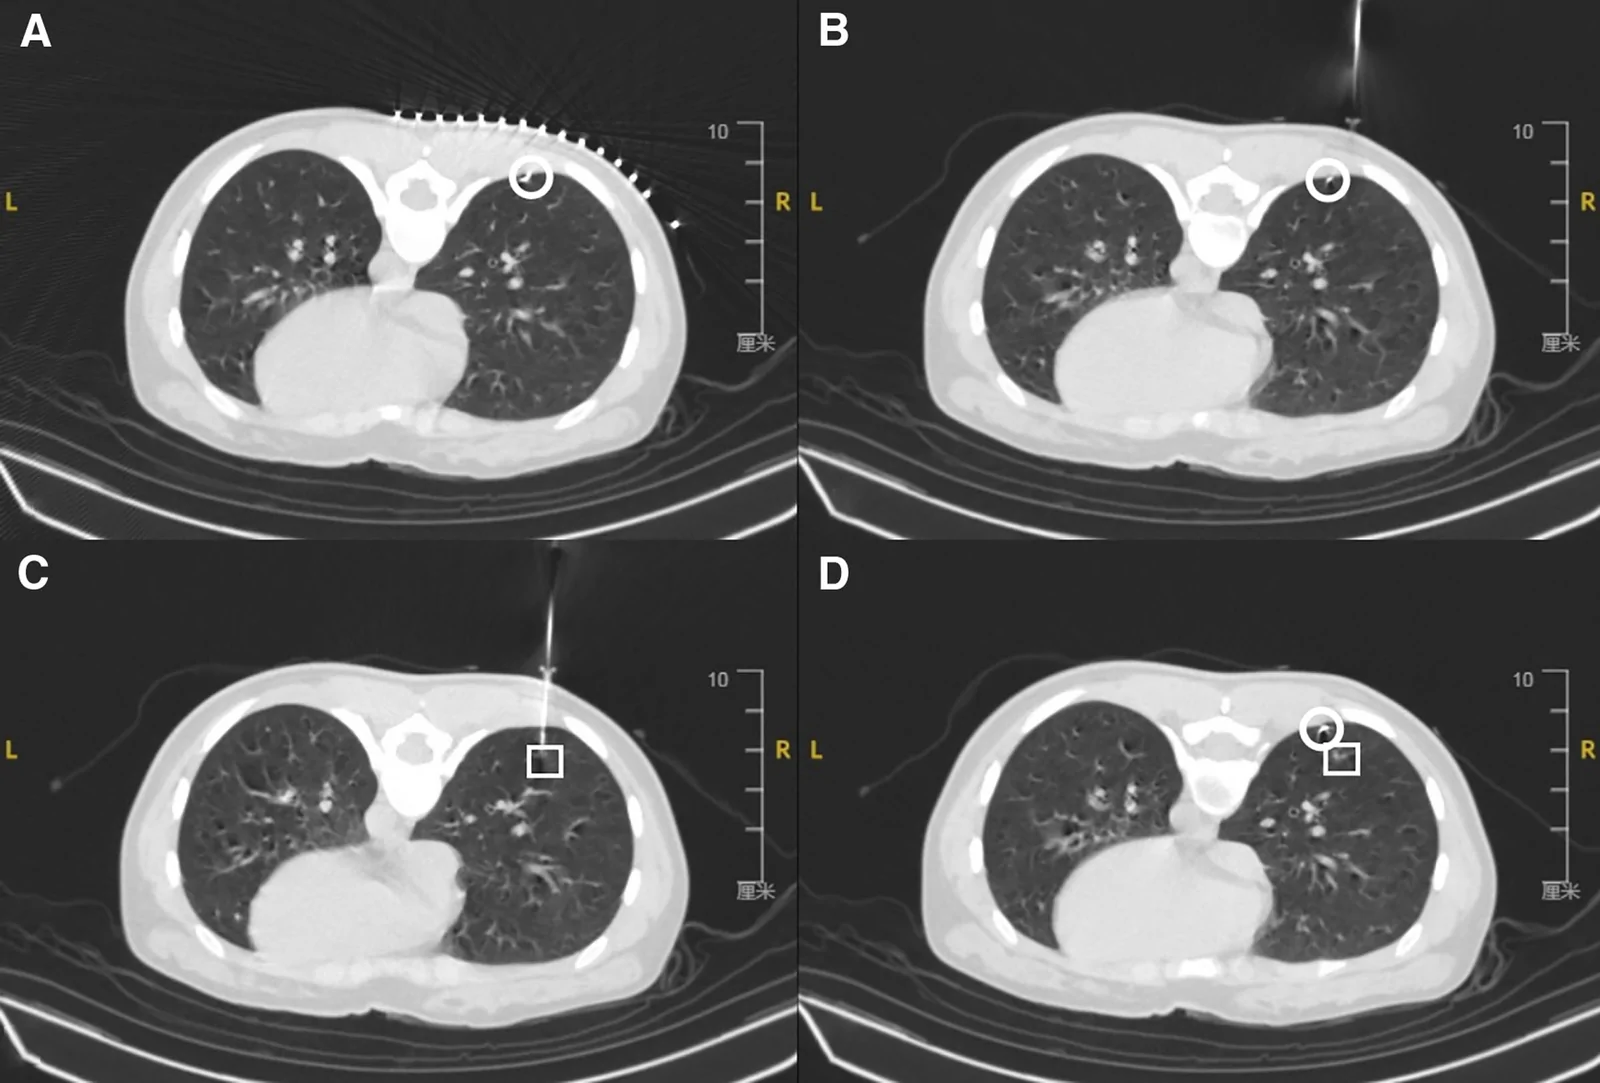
CT-guided preoperative localization of the pulmonary foreign body. (A) A positioning paper was placed on the patient’s back to assist in determining the needle puncture site. (B) After local anesthesia, the localization needle was inserted percutaneously into the thoracic cavity at the identified optimal site. (C) The localization needle tip was advanced near the foreign body within the lung. (D) A localization hookwire was deployed through the needle sheath to secure it in the lung near the foreign body, and the sheath was subsequently removed (white square highlights the localization hookwire, and white circle denotes the sewing needle). CT = computed tomography.
